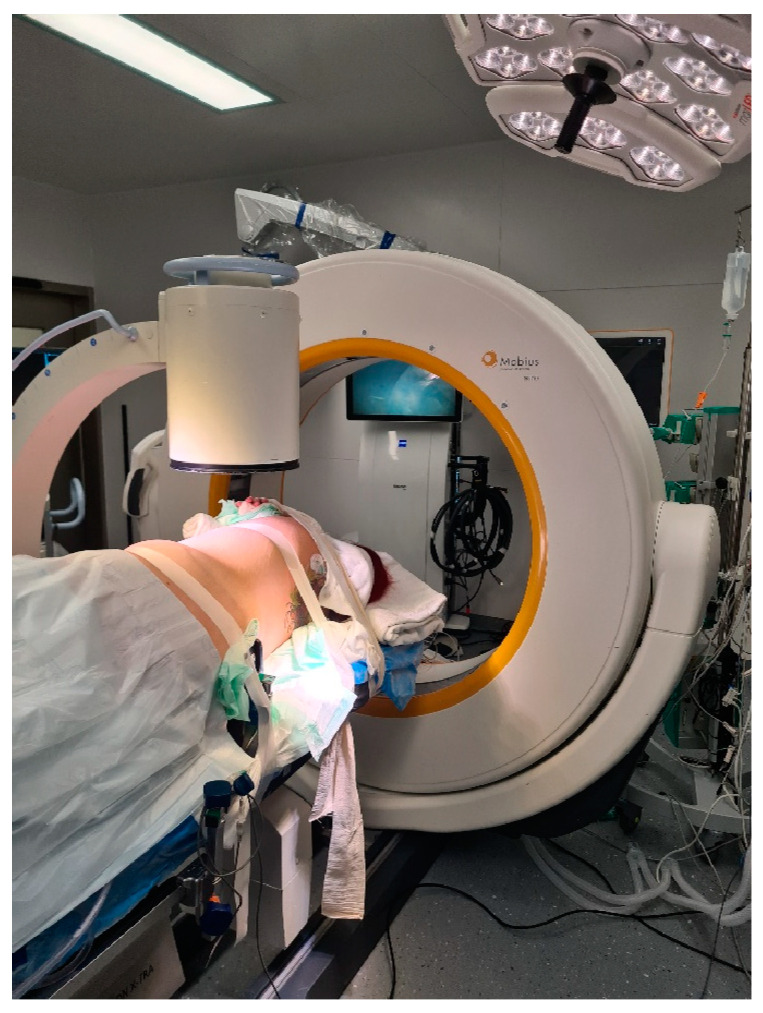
Use of standard C-arm X-ray for level definition prior to skin incision (same as Figure 1).
